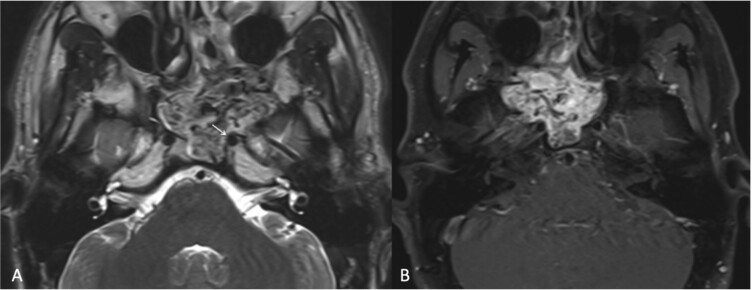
(a) Transaxial T2-weighted MRI image showing a mass with heterogeneous signal intensity and multiple flow voids, along with thinning of the cortical bone overlying the left ICA (arrow). (b) Post-contrast transaxial T1-weighted MRI image demonstrating avid contrast enhancement of the JNA.
